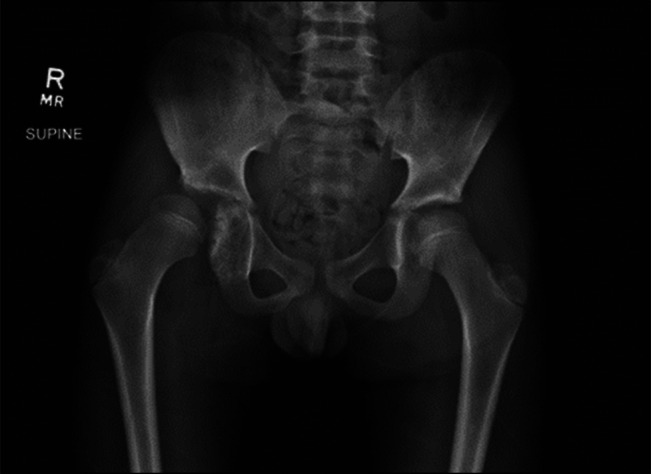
Initial radiograph showing mass lesion in right acetabulum causing subluxation of the right hip and abnormal development of right hip with elevated acetabular index and blunting of lateral sourcil.
